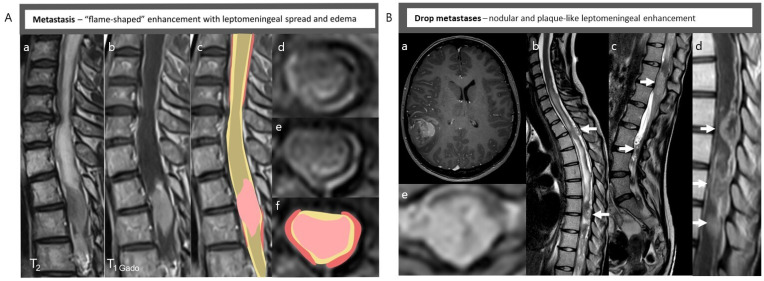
(A) Metastases: (a) Sagittal T2-weighted image showing a hyposignal intramedullary mass with extensive edema; (b) Sagittal T1-contrast-enhanced image showing an intense enhancement with leptomeningeal dissemination; (c) Sagittal pictorial representation; (d,e) Axial T1-contrast-enhanced image; (f) Axial pictorial representation. (B) Drop metastases: (a) Axial T1-contrast-enhanced image showing a posterior temporal glioblastoma; (b,c) Sagittal T2-weighted images of the entire spinal cord showing multiple (arrows) extraxial and intraxial nodule, with extensive intramedullary edema; (d) Sagittal T1-contrast-enhanced image; (e) Axial T1-contrast-enhanced image showing extra/intra axial tumor.
